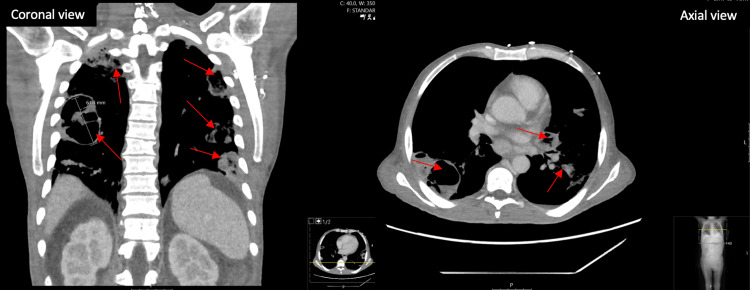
Initial chest CT with contrast in the coronal and axial views showing patchy airspace opacities in the upper lobe and interval development of multiple cavitary lesions Red arrows indicate cavitary lesions.
